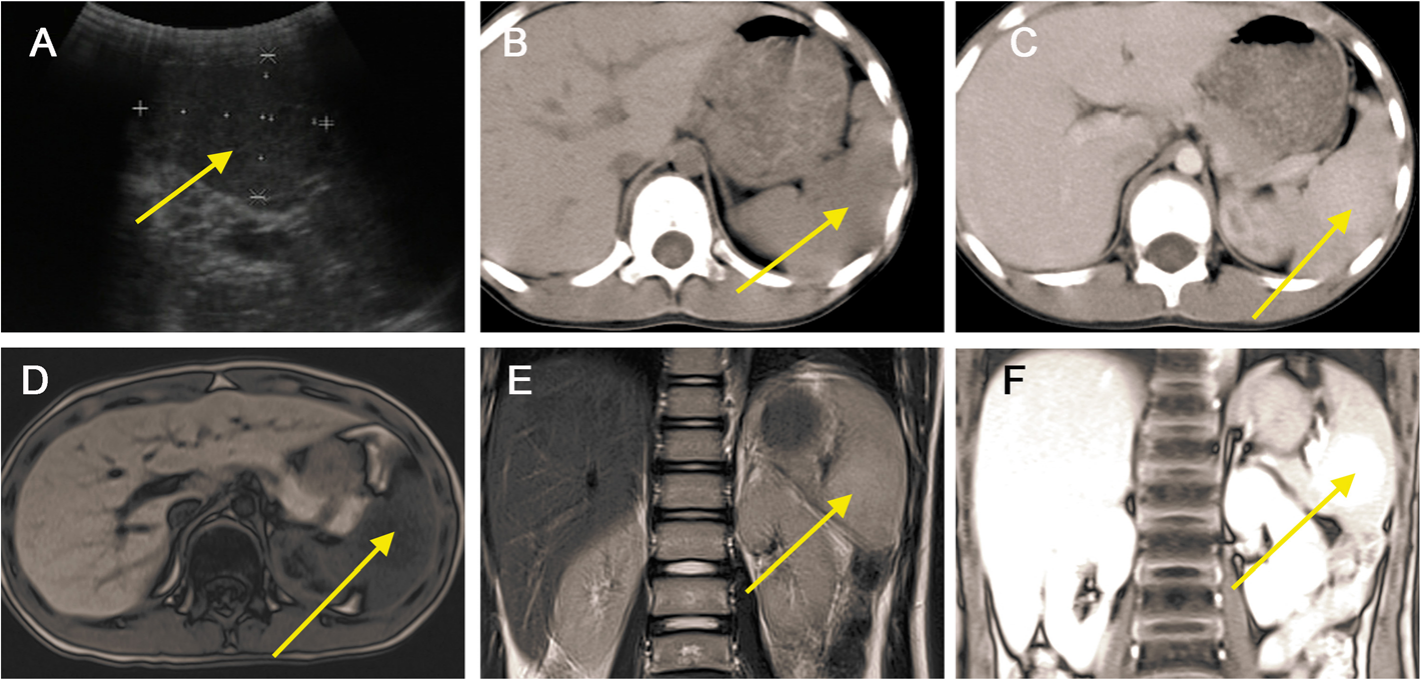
Splenic hamartoma in an 8-year-old boy was found during examination. (A) Abdominal ultrasonography showed an isoechoic oval-shaped mass (*arrow*) in the mid-portion of the spleen; (B) Non-enhanced CT showed a slightly lower-density mass (*arrow*) in the spleen. (C) Contrast-enhanced CT scan shows mild diffuse heterogeneous enhancement in the arterial phase. Magnetic resonance imaging (MRI) showed a hypointense mass (*arrow*) on the T1WI image (D) and a slightly hyperintense mass in the T2WI image (E). (F) The lesion (*arrow*) shows moderate enhancement on gadolinium-enhanced MRI.
